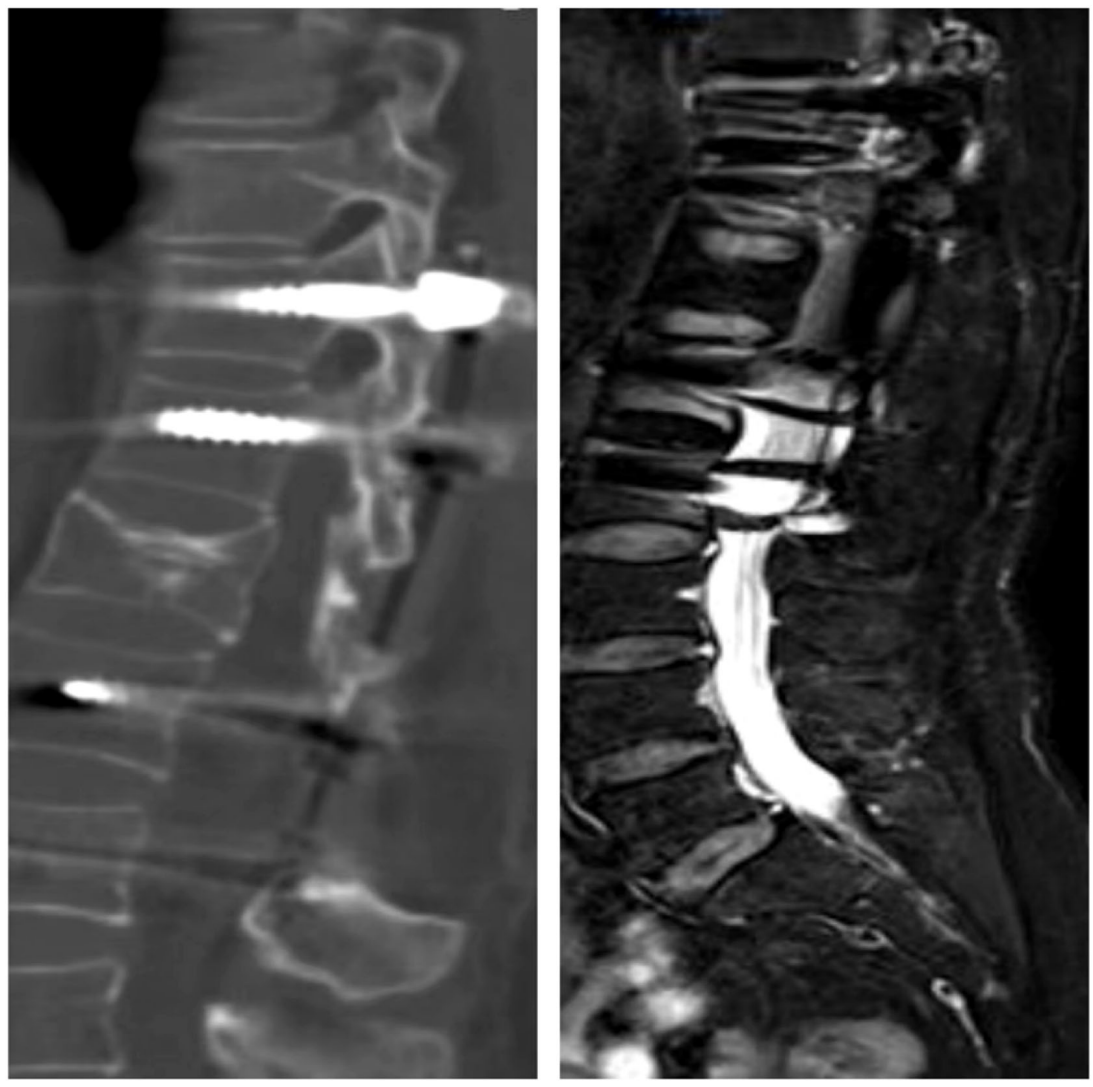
Three-dimensional CT revealed a wedge-shaped deformity of the T12 vertebral body, with no bone fragments within the spinal canal. The L1 vertebral body and its appendages were intact, with no evidence of bone destruction.
